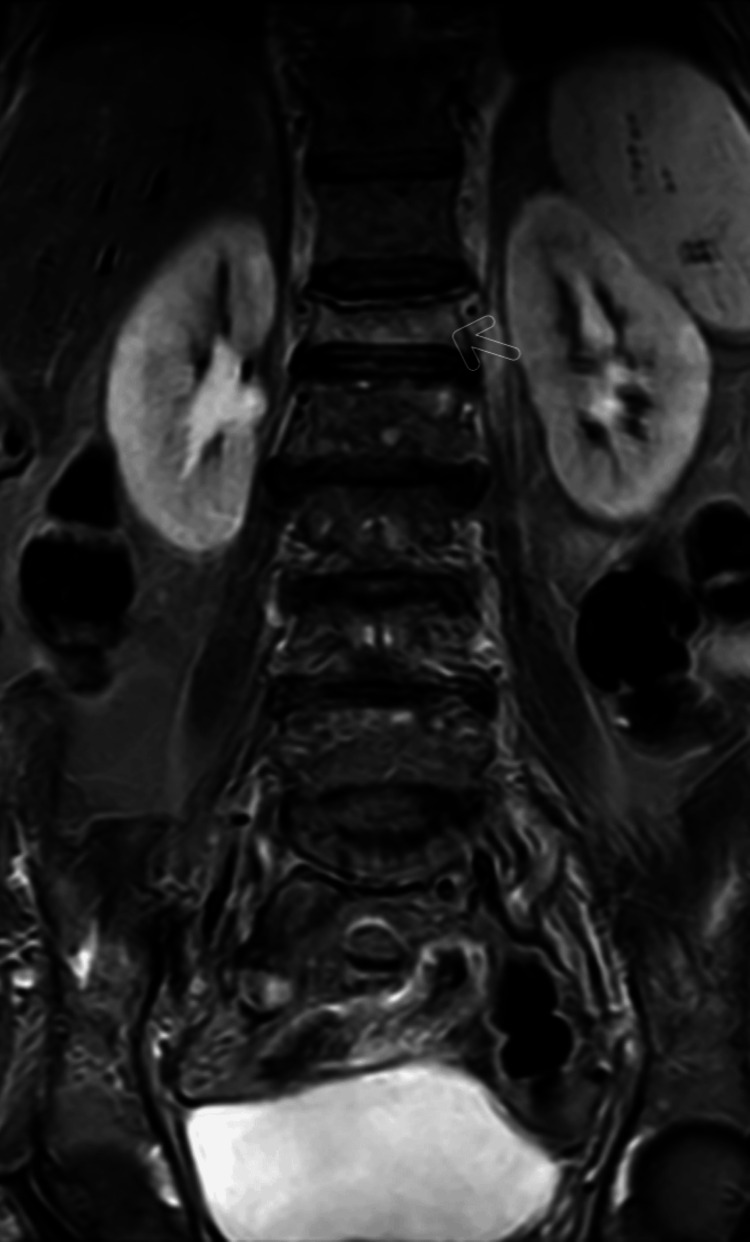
Coronal MRI lumbar spine showing wedge collapse fracture of L1
